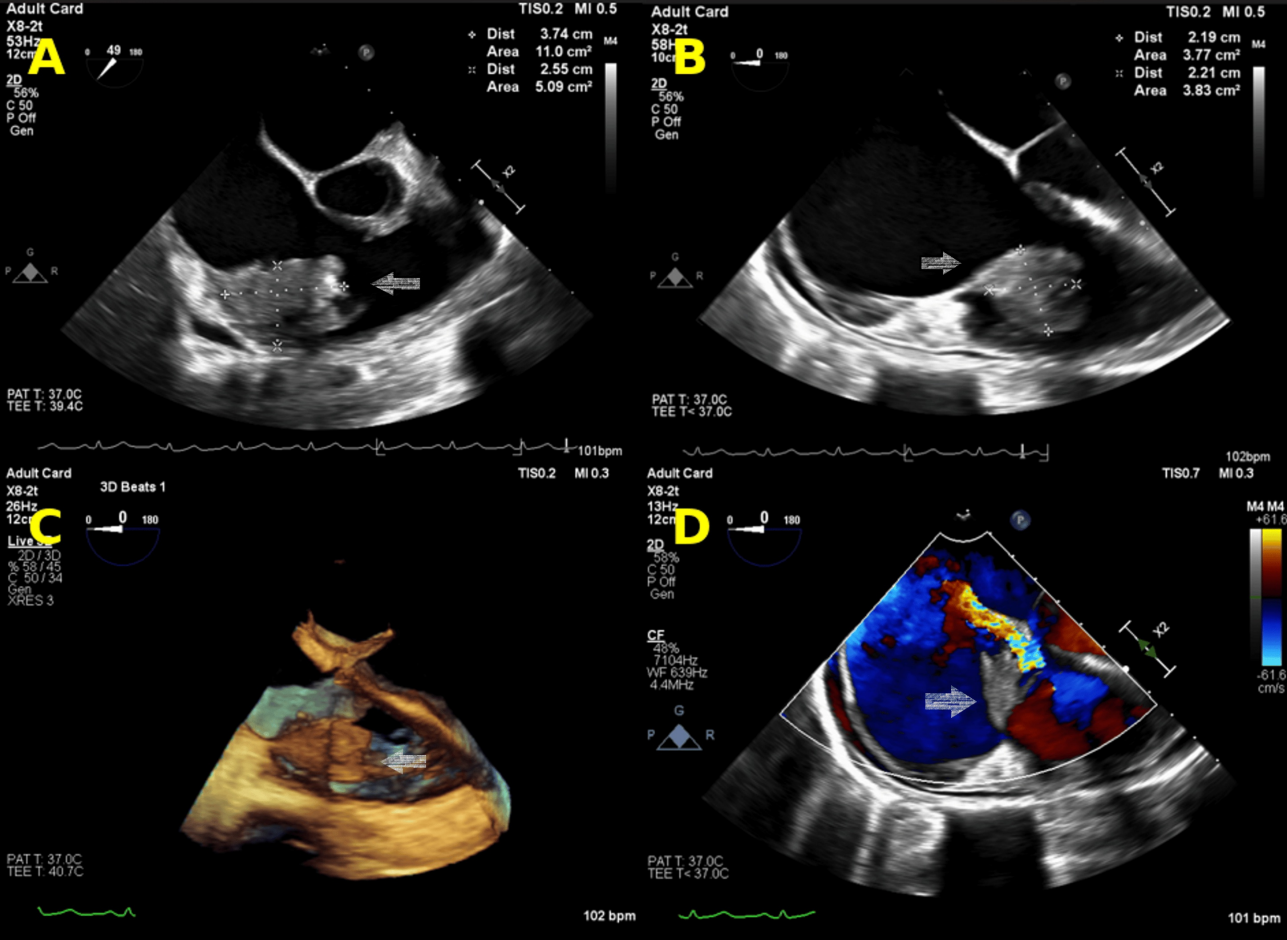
Transesophageal echocardiography (TEE) imaging of the tricuspid valve in infective endocarditis. (A) Midesophageal view at a 49° omniplane angle showing a vegetation measuring 3.7 × 2.6 cm. (B) Midesophageal view at a 0° omniplane angle with vegetation measuring 2.2 × 2.2 cm. (C) 3D echocardiographic image visualizing the large vegetation on the anterior tricuspid valve leaflet. (D) Midesophageal view with color Doppler demonstrating severe eccentric tricuspid regurgitation.
